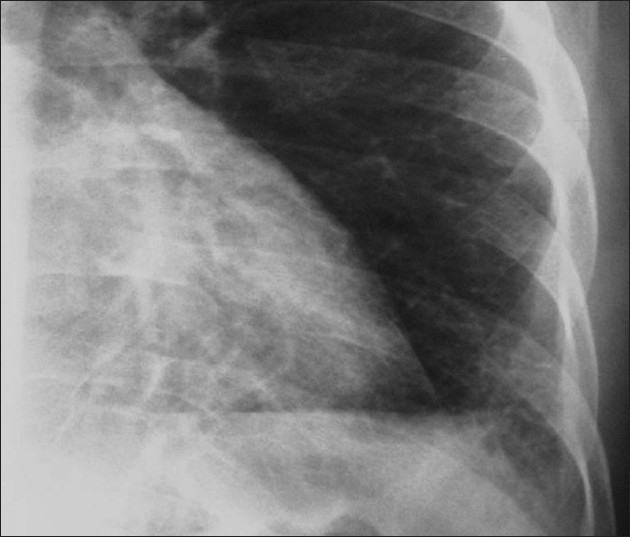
Cryptococcosis. Imaging findings are varied and nonspecific. Reticular chest X-ray or reticulonodular infiltrates are the most common pattern as in this case where a reticulonodular infiltrate involved the left costophrenic angle
